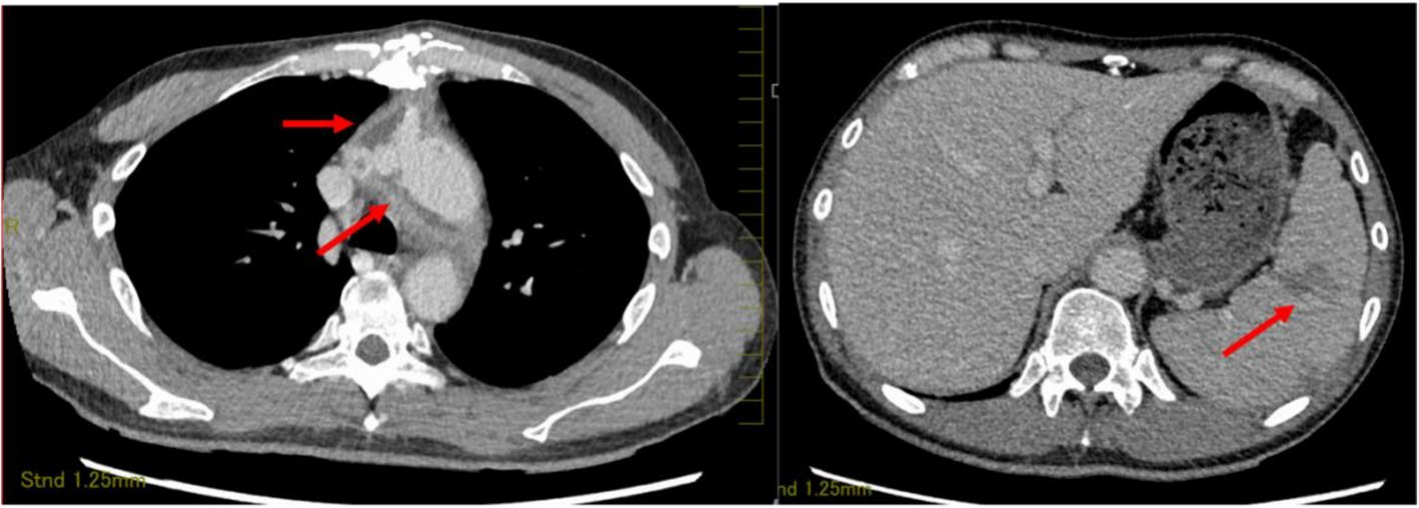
Contrast-enhanced computed tomography of aortic aorta (left), spleen, and liver (right). Contrast computed tomography (CT) revealed fluid correction around the graft vessel (left, arrow), hepatomegaly, splenomegaly (spleen index = 720), and multiple thrombi (right, arrow)
